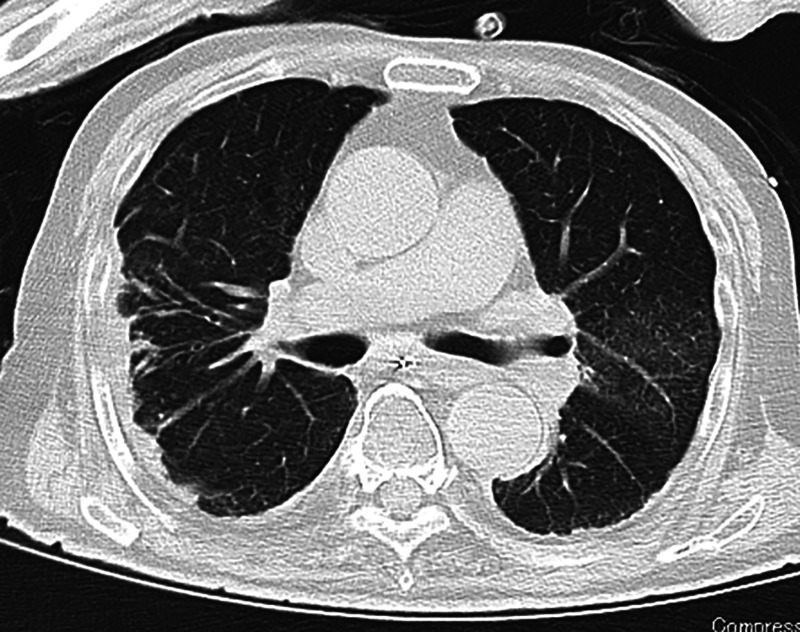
Chest computed tomography (CT) demonstrates multifocal and peripheral ground-glass opacities with predominant consolidation in the mid to lower lung.
